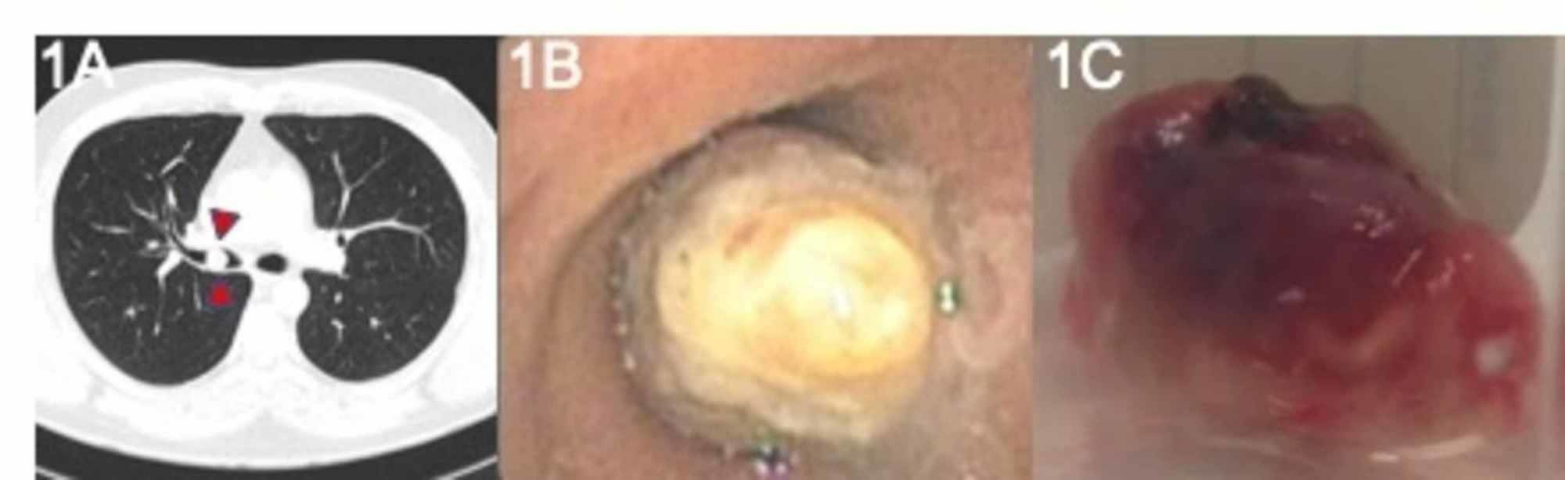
(A) Obstructing mass visualized on CT of the chest (red arrowheads); (B) Obstructing mass visualized in the distal right mainstem bronchus during inspection bronchoscopy; (C) Gross view of mass following resection
